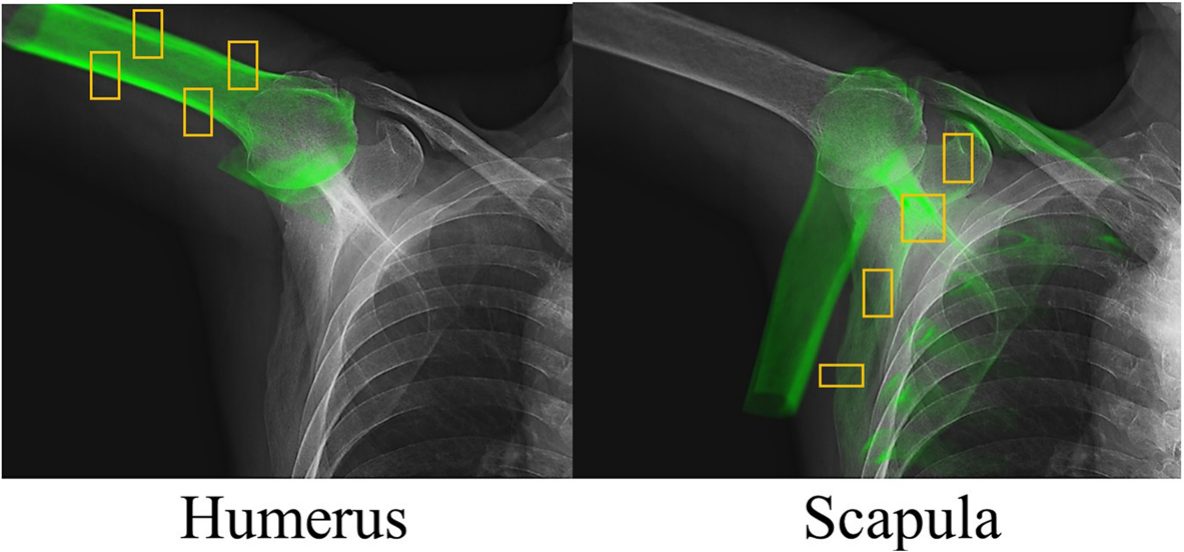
Shoulder computed tomography slices were used to create density-based digitally reconstructed radiographs of the humerus and scapula, which were projected onto the shoulder X-ray images
